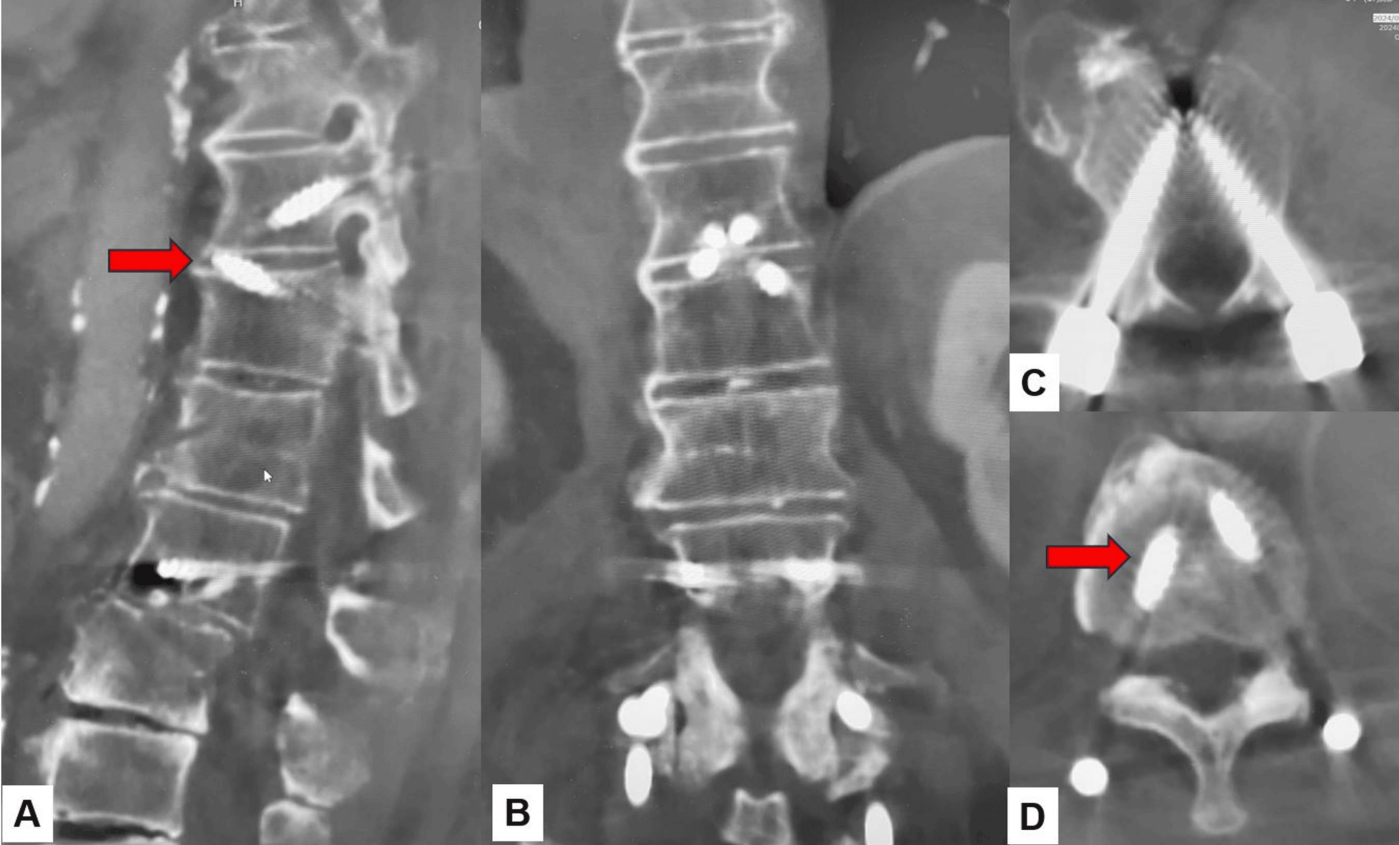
Postoperative CT A: Paramedian reconstruction CT. B: Coronal reconstruction CT. C: Axial CT at T11. D: Axial CT at L2. The transdiscal screws are penetrated into the endplate (red arrows). CT: computed tomography
